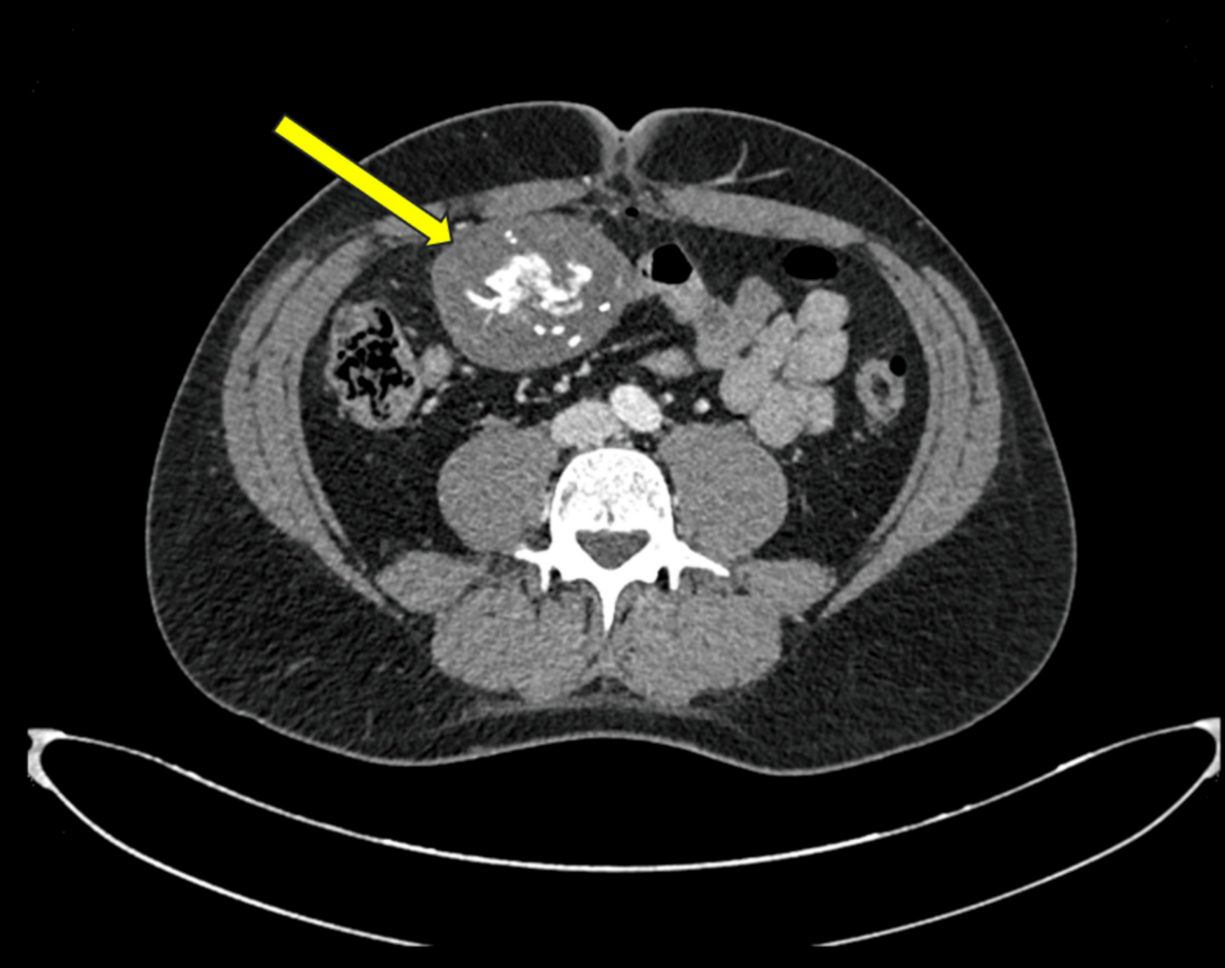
CT scan shows the gossypiboma in the right iliac fossa in axial view. The yellow arrow indicates the gossypiboma.
